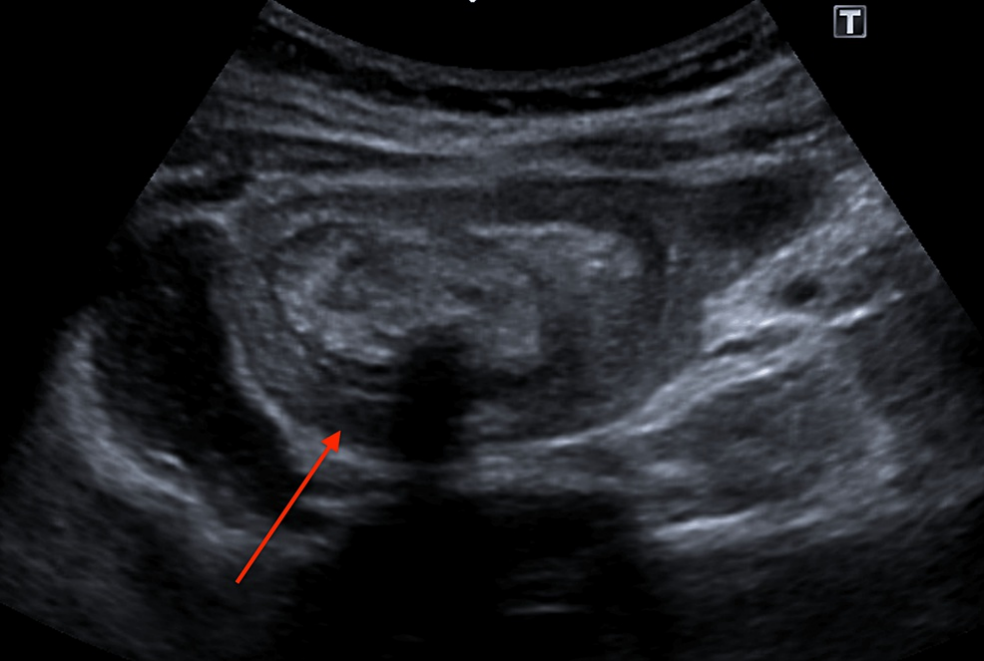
Ultrasound scan six years prior (aged 13) demonstrating target sign characteristic of intussusception
